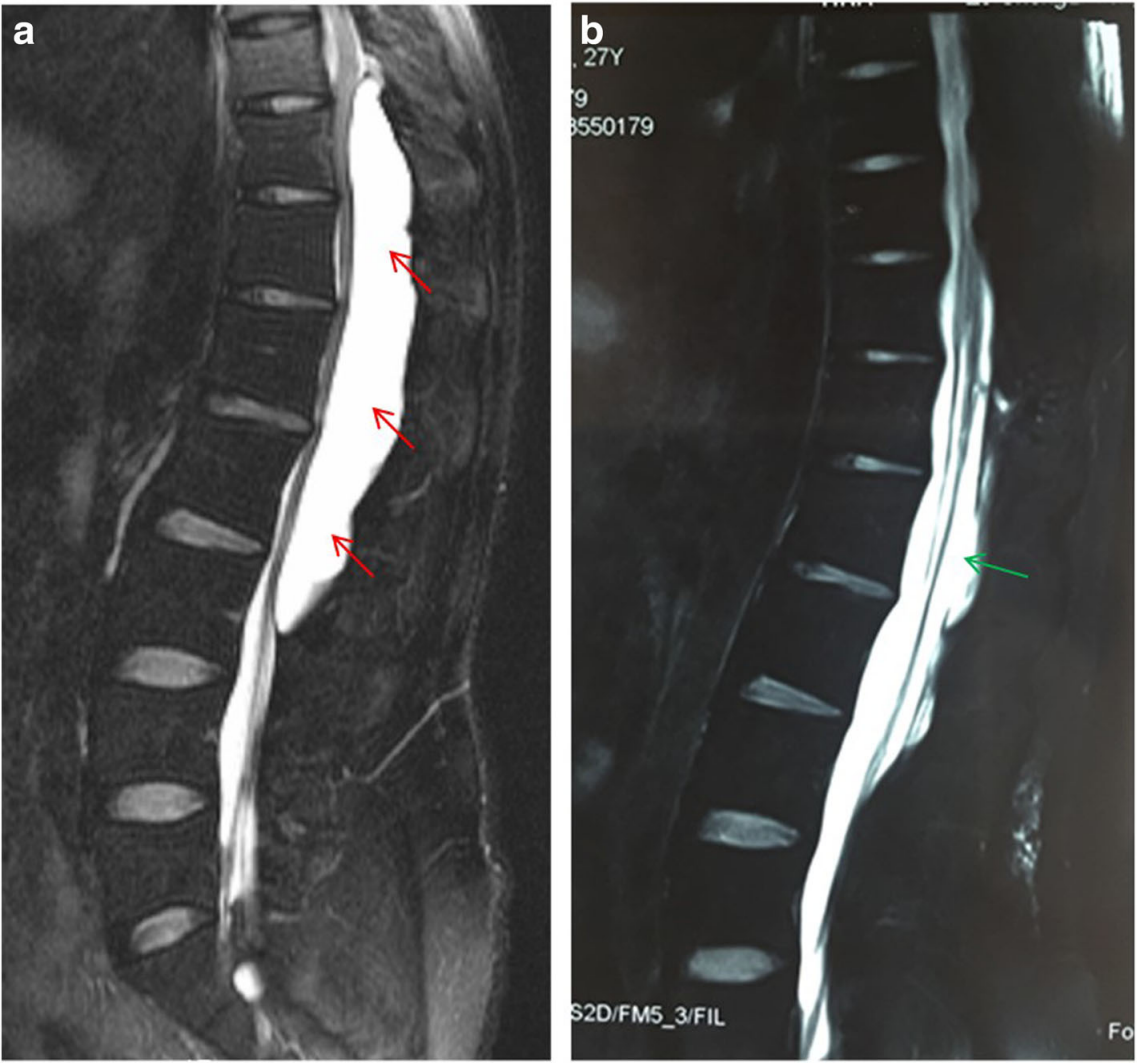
a The pre-operative magnetic resonance imaging (red arrows); b The 3-month follow-up magnetic resonance imaging: there was no recurrence of the cyst (green arrows)
